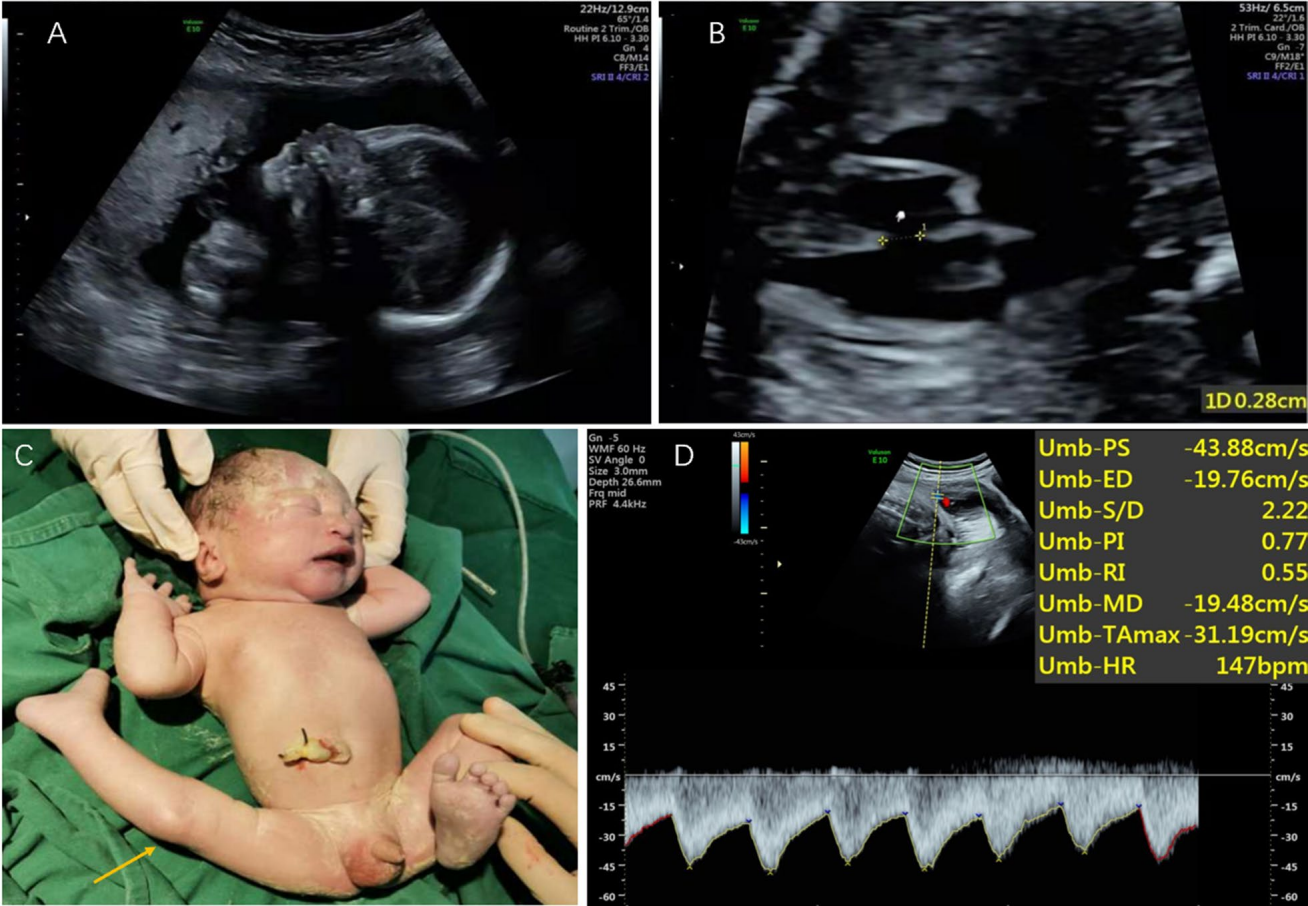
Foetal ultrasound at 24 weeks and abnormal newborn detections: absence of nasal bone (A) and ventricular septal defect (B). The right knee joint of the newborn was dislocated (C). Colour ultrasound indicated congenital heart malformation: ventricular septal defect; atrial septal defect (muscle) (D)
